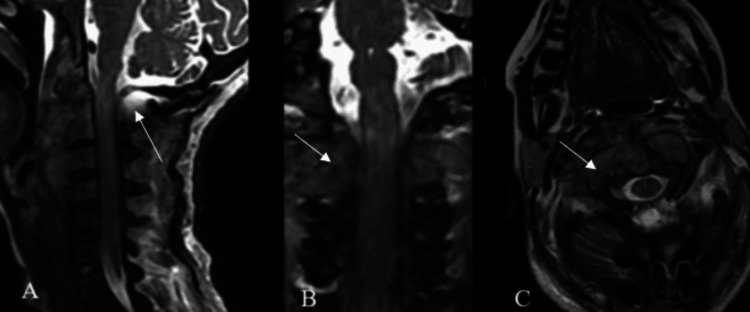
Follow-up postoperative T2 MRI A. Sagittal view: Follow-up MRI at a later postoperative stage shows maintained cervical spine alignment (10°) and sustained decompression at the bulbomedullary junction. B. Coronal view: Craniocaudal hyperintensities extending from C1 to C3 reflect chronic white matter damage from prior compression, now within a decompressed cervical spinal cord. C. Axial view at C1 level: Evidence of surgical decompression, with cerebrospinal fluid (CSF) hyperintensity surrounding the spinal cord. The posterior arch of C1 is absent, consistent with prior surgical drilling.
